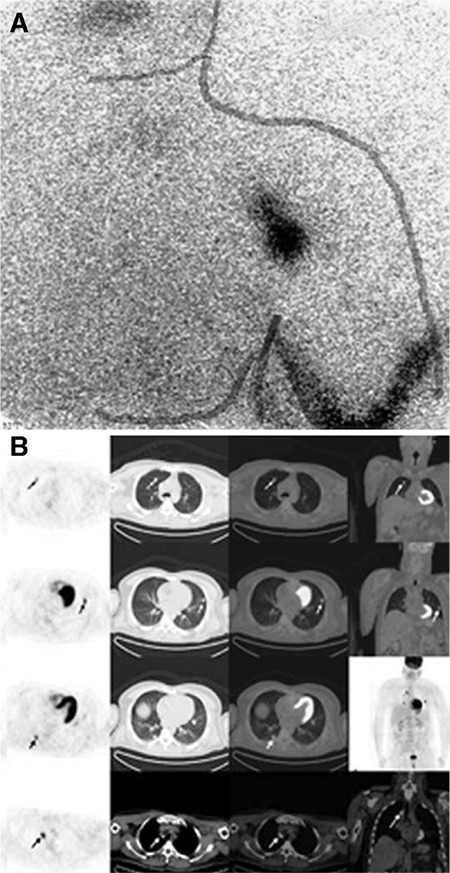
Preoperative lymphoscintigraphy image of a patient with a positive axillary sentinel lymph nodes biopsy (A) along with metastatic lun nodules and mediastinal lymph nodes of the same patient in PET/CT scan performed within the 1^st^ postoperative year (B)
